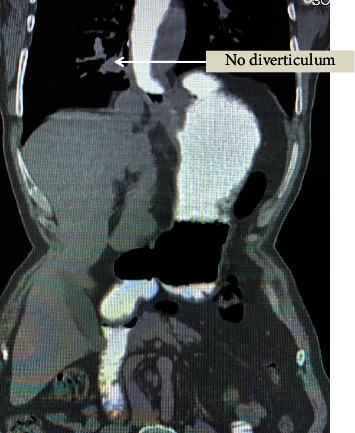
Immediate postoperative CT showing no outpouching or leak from oesophagus.
